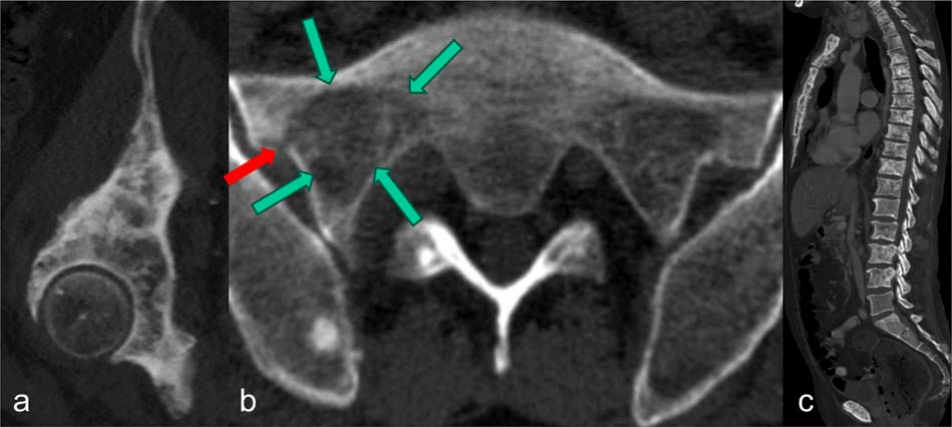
In the sagittal reformation of the right hip (a), in the axial CT section of the right ilium (b) and in the sagittal reformation of the spine (c) osteoblastic metastases of the known prostate carcinoma are visible. In (b), there is also an osseous defect zone (marked with green arrows) in the right lateral mass of the sacrum with a pathological fracture (marked with red arrow) in the area of the lateral cortical border.
